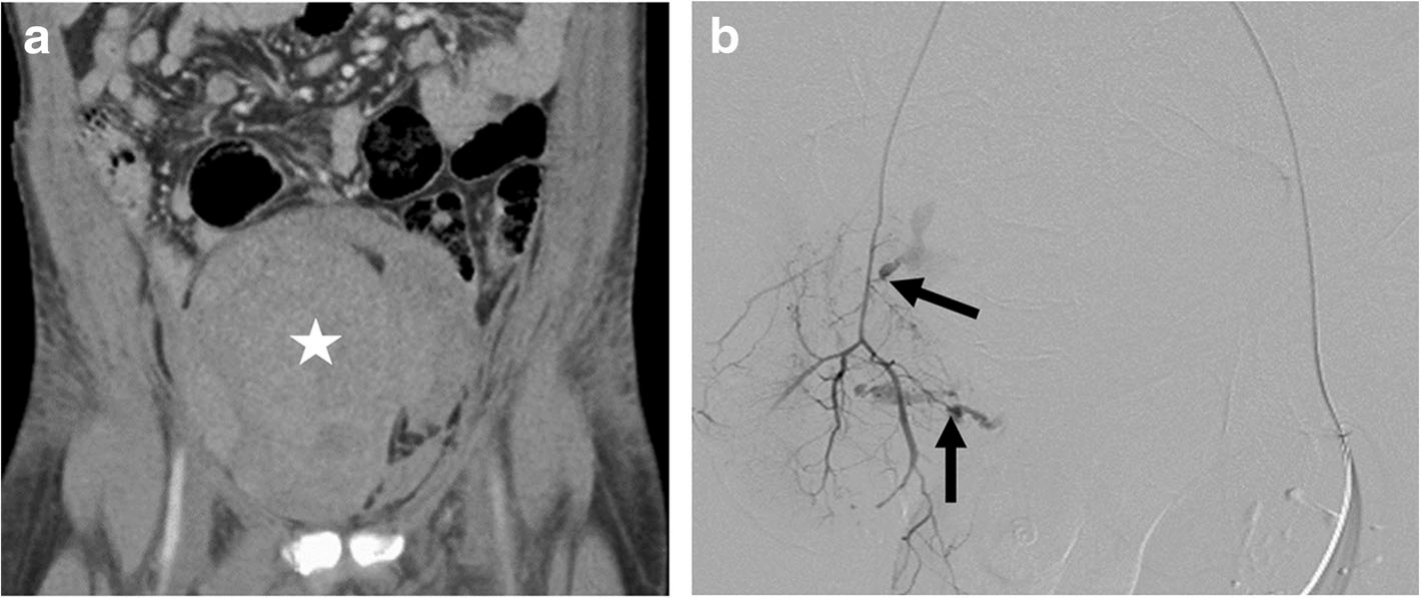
a. Coronal reconstruction of a negative CTA. A large extraperitoneal hematoma (star) is seen in the pelvis. No active extravasation of contrast or abnormal vessel was seen on CTA. b. Posterior-anterior projection digital subtraction angiogram of the obturator branch of the anterior division of the right internal iliac artery. This was performed using an end-hole catheter inserted from a left common femoral artery access sheath. Active hemorrhage is seen from two branch arteries (black arrows)
